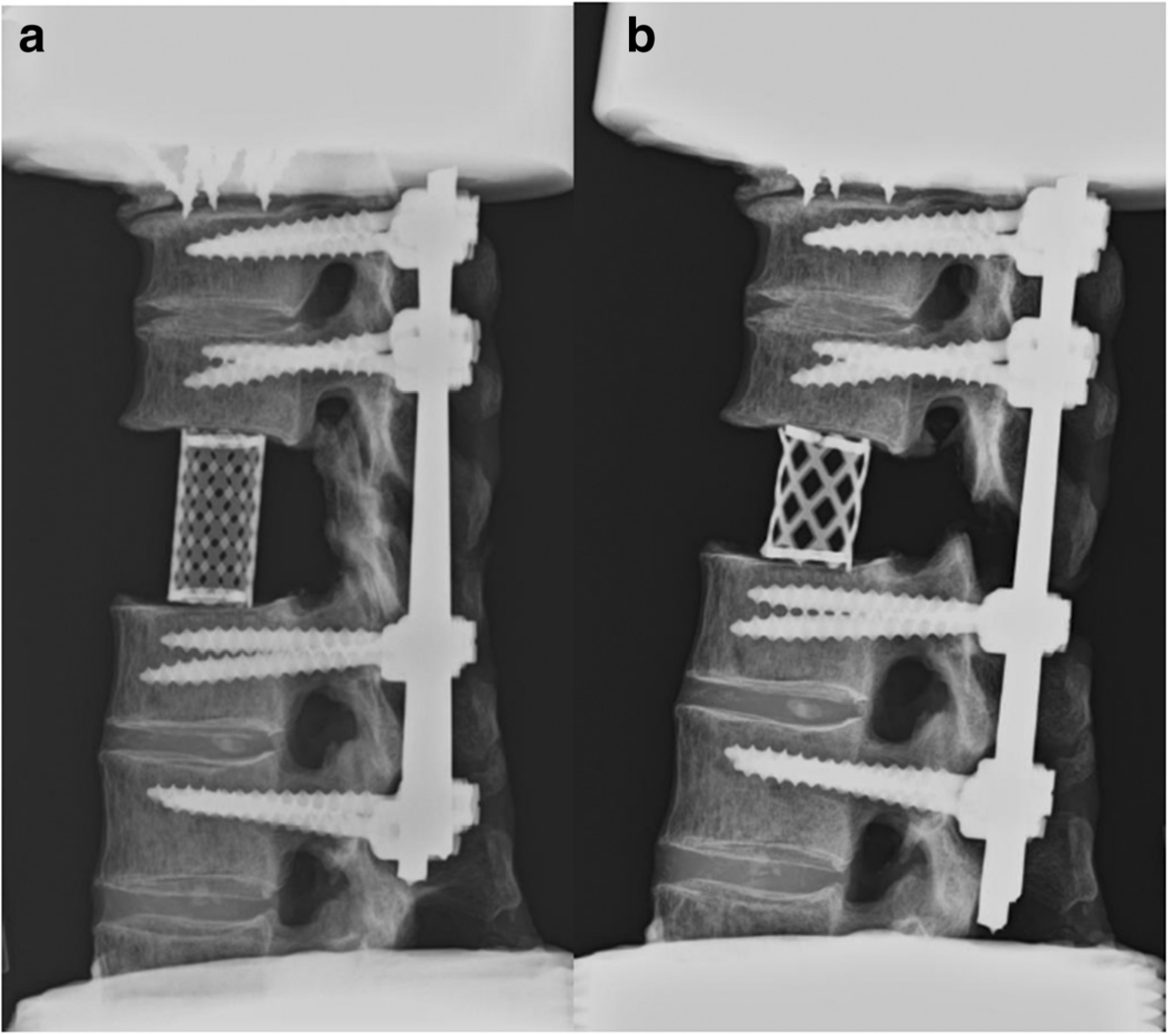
Lateral radiographs of the two reconstruction models. a After corpectomy, reconstruction with anterior cage and posterior instrumentation. b After total spondylectomy, reconstruction with anterior 10-mm shorter cage and posterior instrumentation
